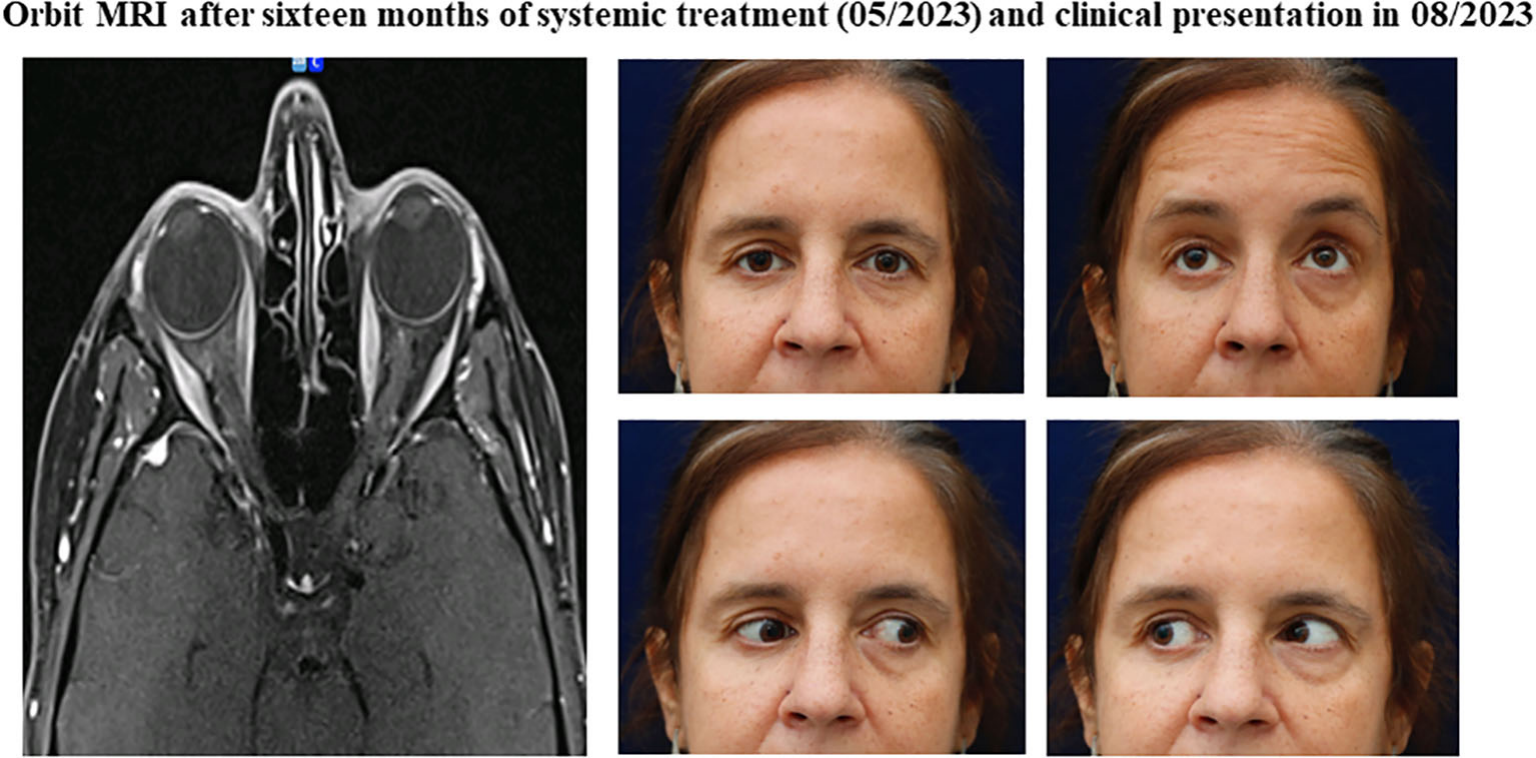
Orbital findings after sixteen months of systemic treatment and clinical presentation in 08/2023. (Orbit Imaging) Orbit axial and coronal T1 MRI showing imaging improvement in the orbital region, marked by permeabilization of bilateral intraorbital fat. (Clinical pictures) Significant clinical improvement in ocular movement restrictions, with only partial limitation remaining on right adduction.
